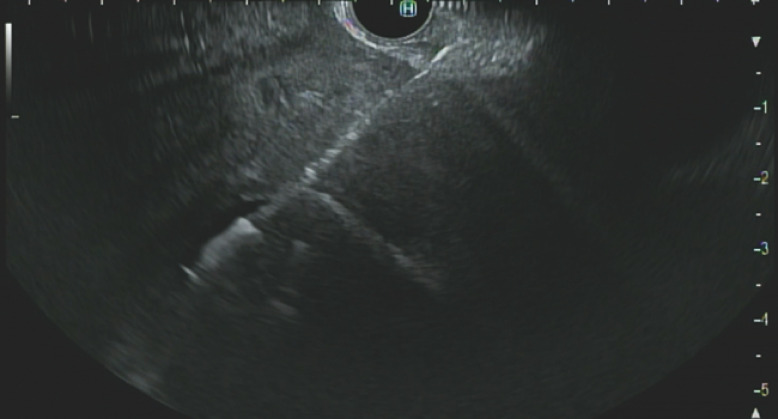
Endoscopic ultrasound (EUS) image during EUS-guided cystoduodenostomy.
